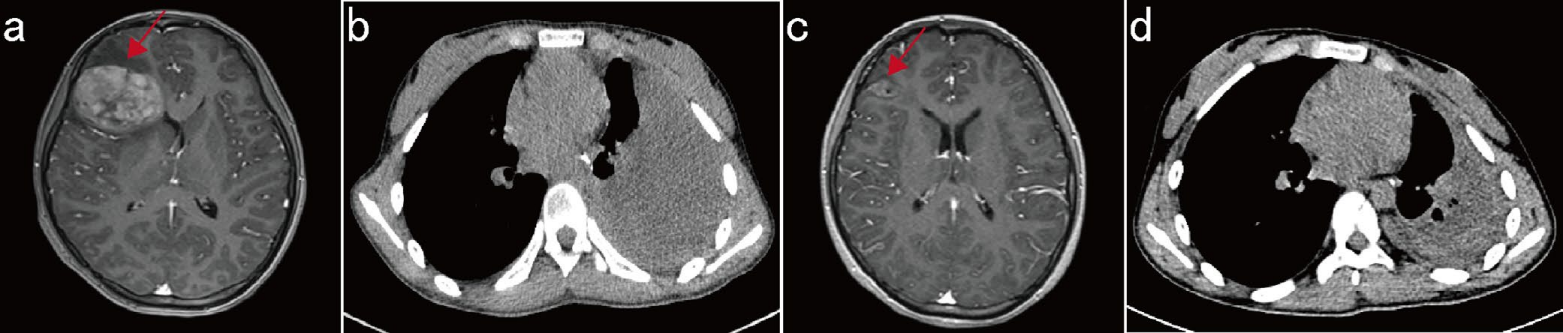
Radiographic images before and after the initiation of ALK‐targeted therapy. Cranial MRI (2024/9/14) revealed a 53 × 37 mm cranial mass (a), and a heterogeneous, slightly hyperdense mass in the left thoracic cavity was detected via a CT scan (b). After 1 month of alectinib therapy, imaging examinations revealed an obvious decrease in the size of the brain metastasis (c). The chest lesion was significantly smaller in size (d).
